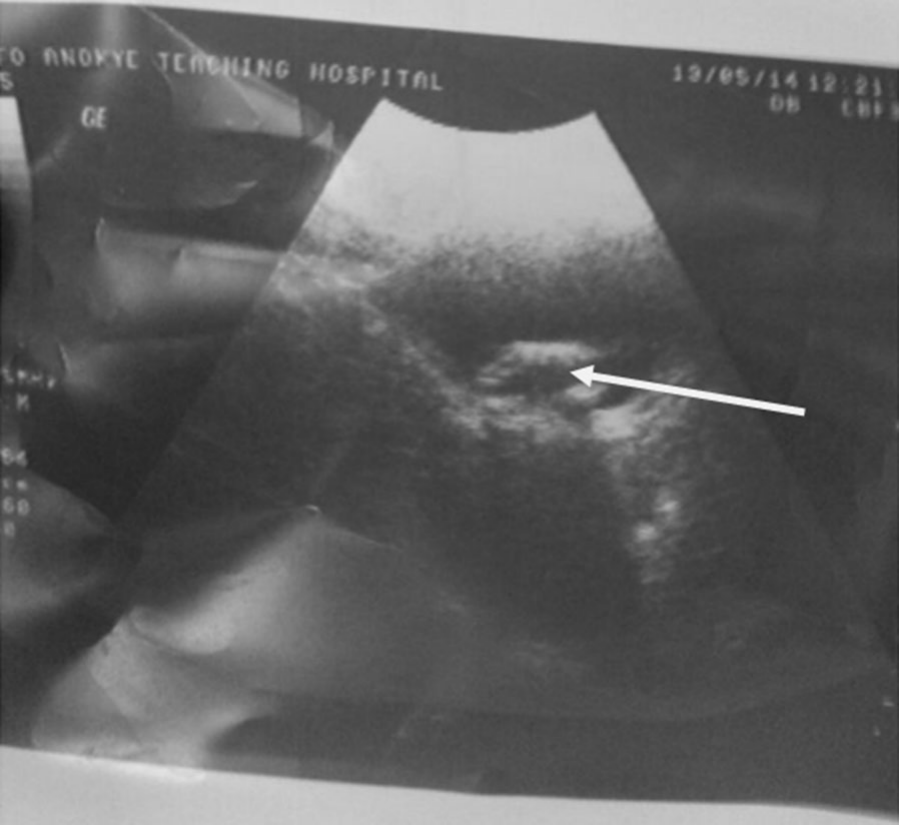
Abdominal ultrasonography showing the intrauterine contraceptive device as a bladder calculus (*arrowed*)
